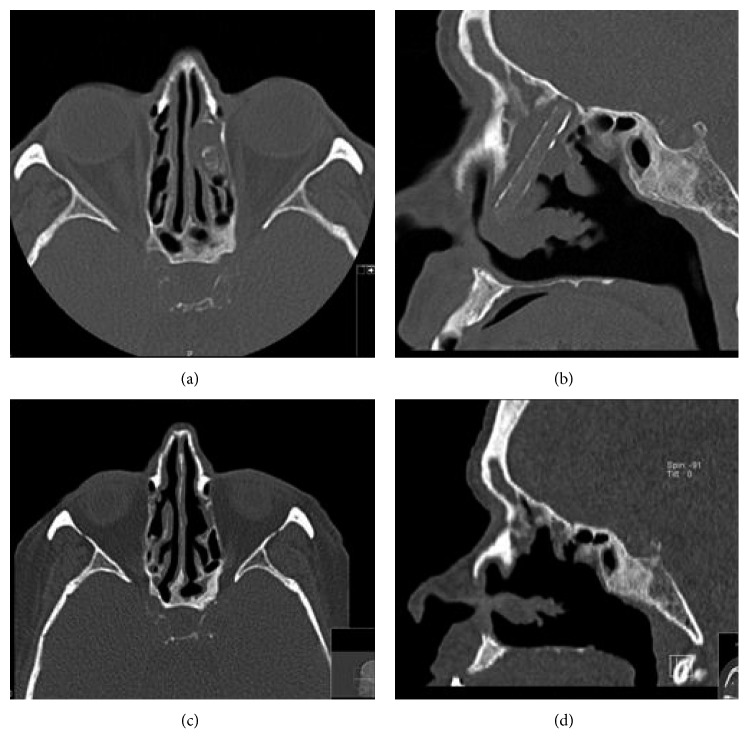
(a, b) Axial and sagittal cuts of a paranasal CT scan showing cylindrical foreign body, in the left nasal fossa and ethmoid sinus; (c, d) coronal and sagittal CT scans 6 months after FESS, with removal of the foreign body.
